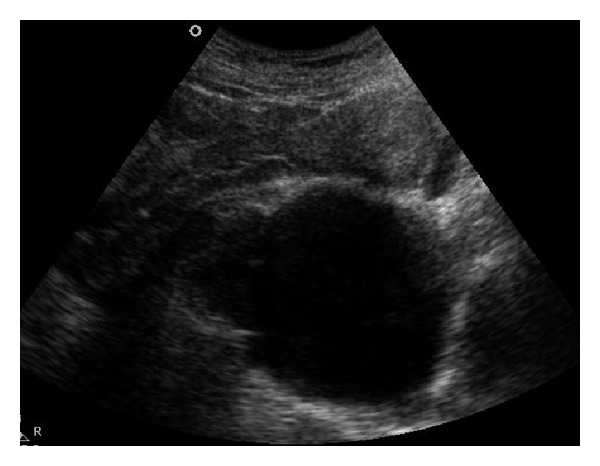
Sagittal ultrasound image of the pelvic area showing a thin-walled cystic lesion compressing the uterus on the posterior.
